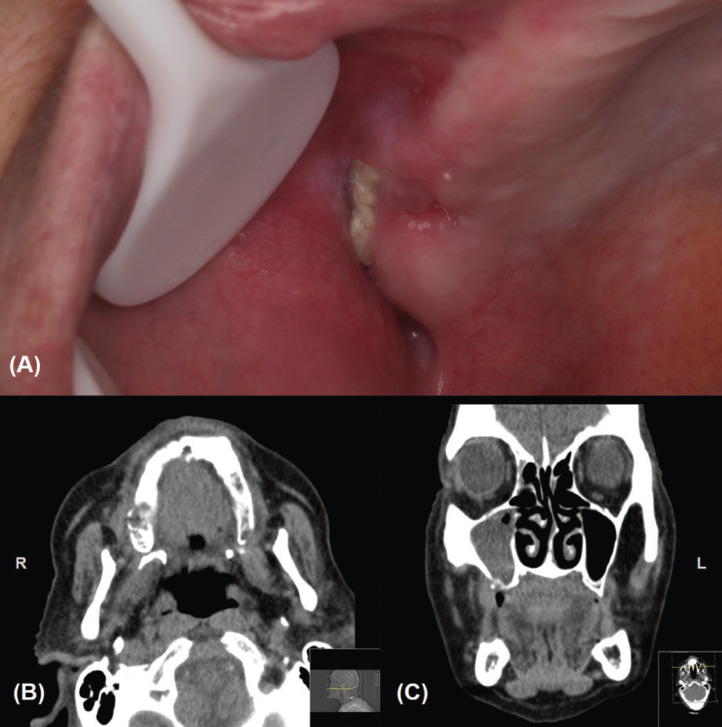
Clinical presentation of a non-healing ulcer with bone exposure compatible with a lenvatinib-related osteonecrosis of the maxilla (A); CT scan showing bone rarefaction of maxillary posterior and right alveolar process (B and C).
